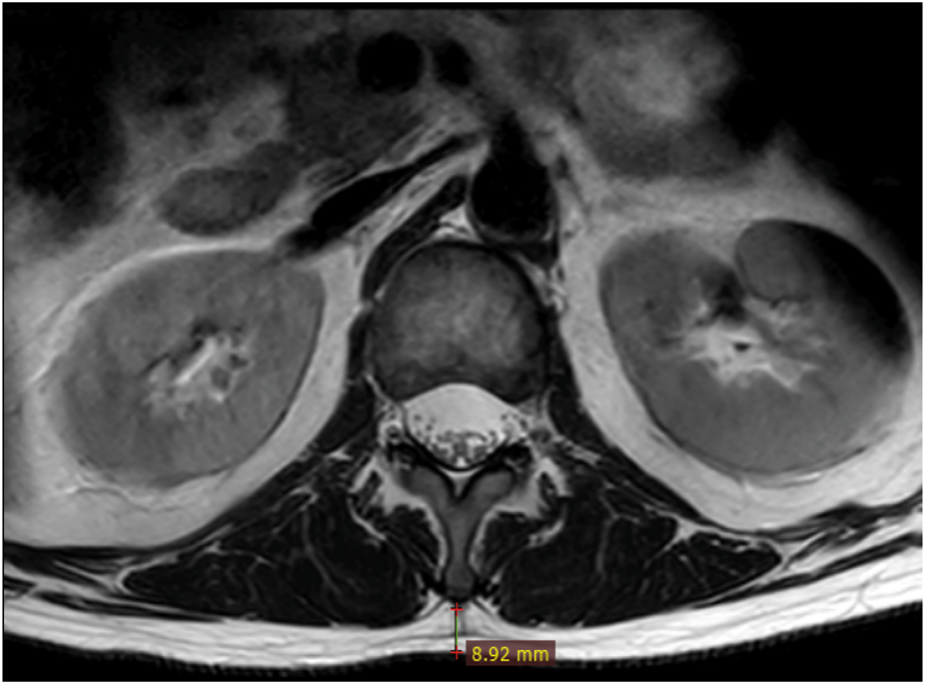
Measurement technique of SFTT(L1/2). At the L1/2 level on T2-weighted axial lumbar MRI, the vertical distance from the L1 spinous process to the skin was SFTT(L1/2).
